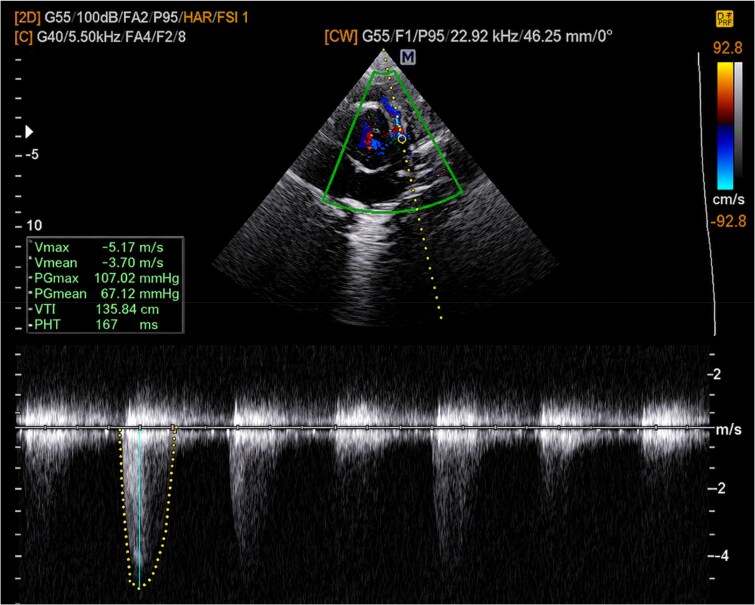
Continuous-wave Doppler echocardiography across the right ventricular outflow tract, obtained during exercise (stress echocardiography), demonstrates a high-velocity systolic jet (*V*max 5.17 m/s) with peak and mean pressure gradients of 107 and 67 mmHg, respectively, confirming severe right ventricular outflow tract obstruction secondary to an interventricular septal hydatid cyst mechanical effect.
